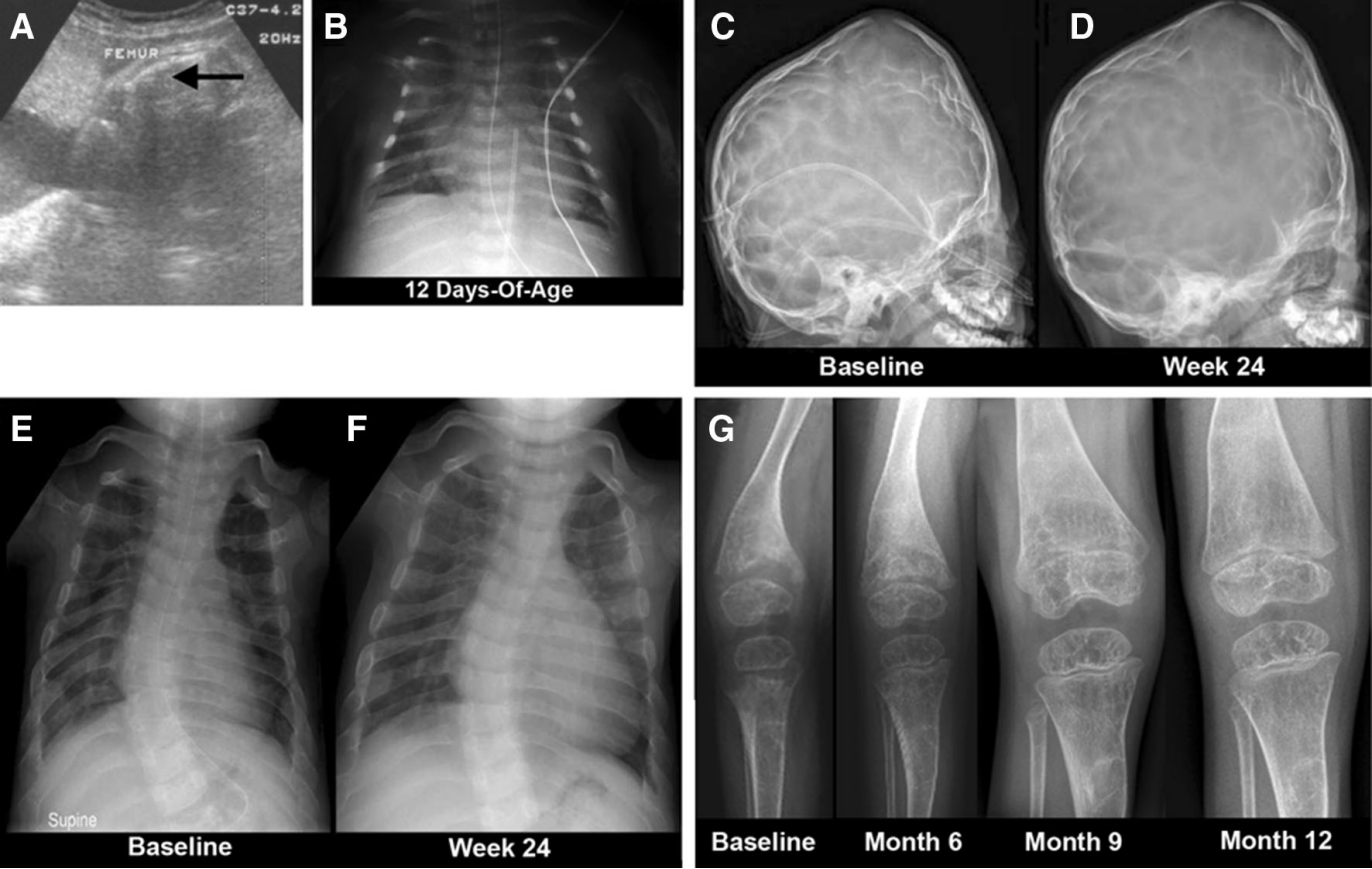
Efficacy of asfotase alfa treatment in a 36-month-old girl (at therapy baseline) with life-threatening HPP. a She has a short, bowed femur detected in utero by ultrasound. b At 12 days-of-age, her chest radiograph showed thin, osteopenic ribs with lytic areas and fractures. c, d Images of the skull before and after 24 weeks of ENB-0040 treatment. Note the severe pan-suture closure, including a marked increase in “digital” markings (“beaten-copper” appearance). e The ribs at baseline were osteopenic and had fracture deformities with thin cortices. f By week 24 of treatment, the ribs were wider and better mineralized with sharper cortical margins and less deformity. g Improvement of the rickets with therapy is apparent. Images taken for the online supplementary data in [9] and reproduced with permission from The New England Journal of Medicine
